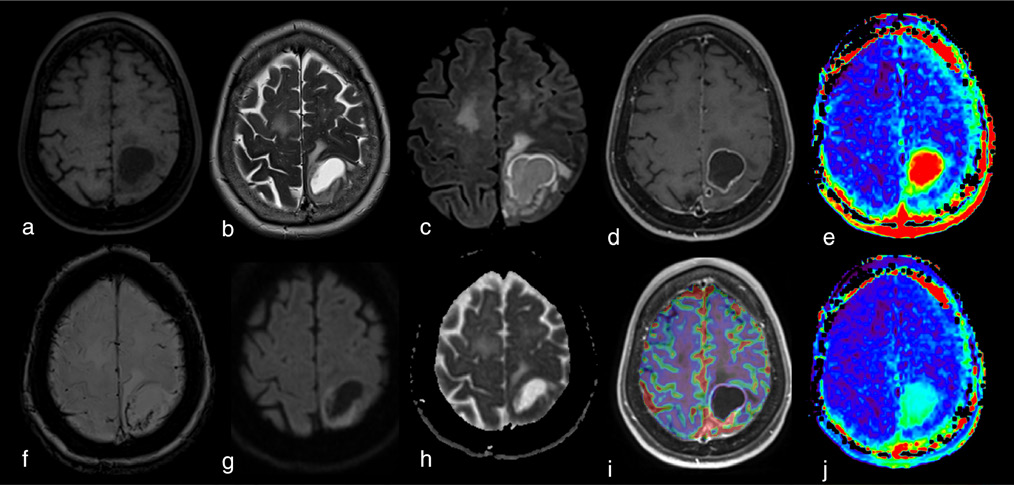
Example of a high-grade glioma (Glioblastoma, IDH-wildtype, WHO grade 4) studied with an MRI multimodal imaging protocol that includes APTw imaging before (e) and after (j) fluid suppression. Notice how the cystic component of the tumor has a high signal intensity (red) in E and lower signal intensity (green) after the fluid suppression. In contrast, tissular components in the posterior part of the lesion maintain their hyperintensity after fluid suppression, suggesting malignancy. (a) three-dimensional T1-weighted spin echo sequence (3D T1w SE). (b)T2 weighted (T2w) image. (c) 3D Fluid attenuated inversion recovery (FLAIR) weighted sequence in the axial plane. (d) 3D T1w SE sequence in the axial plane after contrast injection (3D T1w SE injected). (e) APTw map. (f) susceptibility weighted imaging (SWI). (g) axial diffusion weighted imaging (DWI), b value of 1000 s/mm^2^. H: Apparent Diffusion Coefficient (ADC) map. I: relative Cerebral Brain Volume (rCBV) map after leakage correction superposed to 3D T1w SE injected sequence. J: Fluid-suppressed APTw image (F.S. APTw). All APTw images displayed in this manuscript were acquired at 3 T Skyra or Prisma scanner (Siemens Healthineers, Erlangen, Germany) with a 3D snapshot-GRE sequence^
